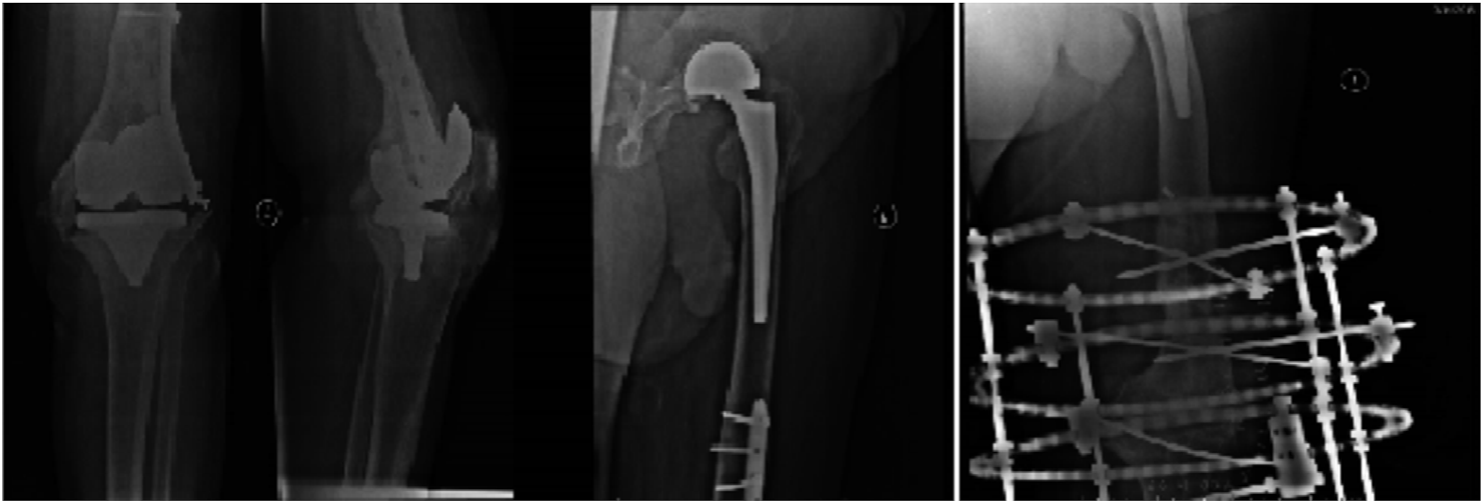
X-ray of the fixation of the periprosthetic fracture of the infected loose left knee prosthesis, also showing the ipsilateral uncemented hip bipolar prosthesis. Knee arthrodesis by Ilizarov external fixator was performed later.
